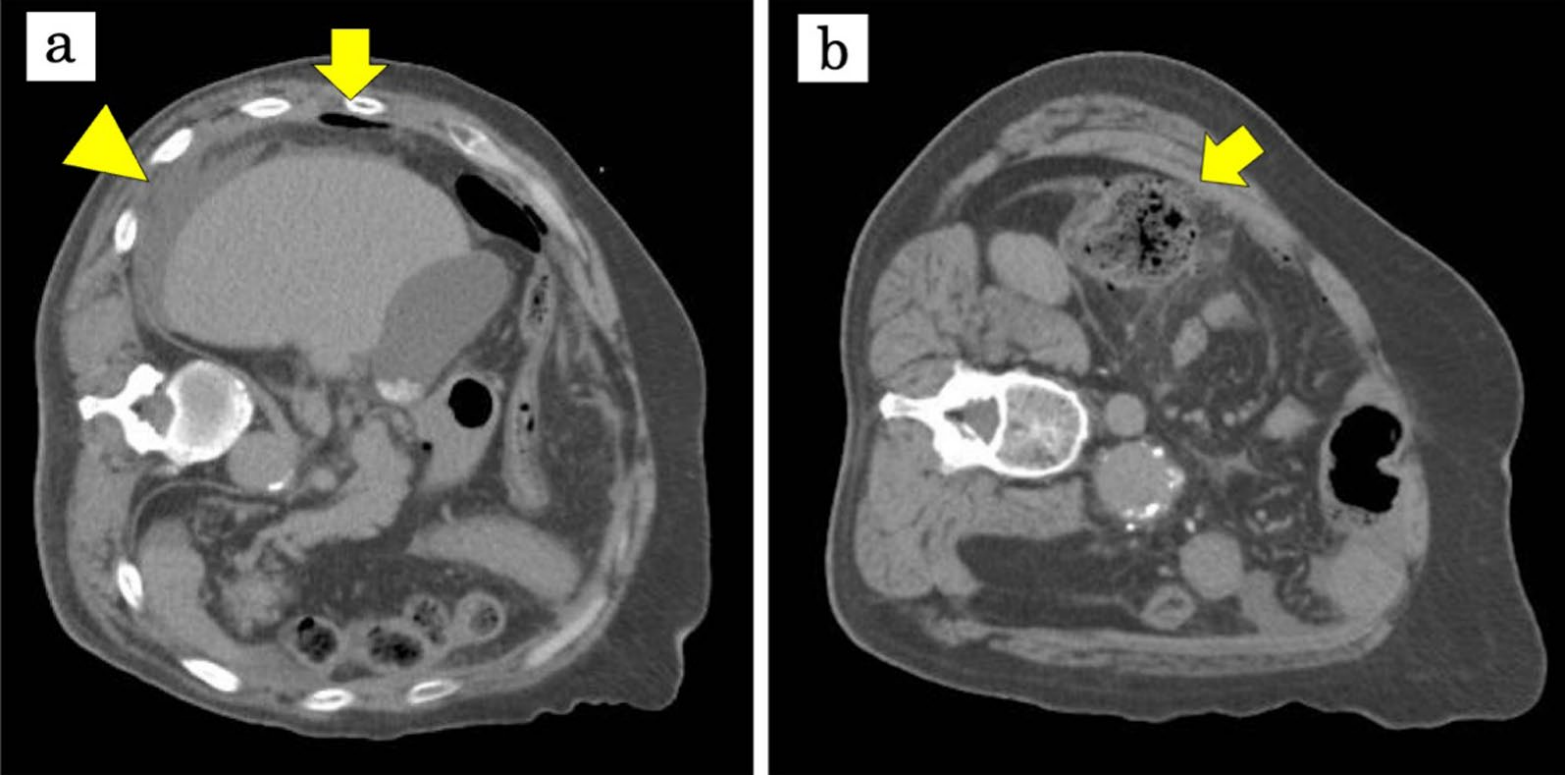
Abdominal computed tomography. During CT examination, the patient could not lie in the supine position due to severe abdominal pain and was scanned on the left decubitus position. CT revealed excessive free air (arrow) and ascites including stool below the liver (arrowhead) (a). The perforation (arrow) was on the proximal side of the mass (b). There are no apparent diverticula in CT images
